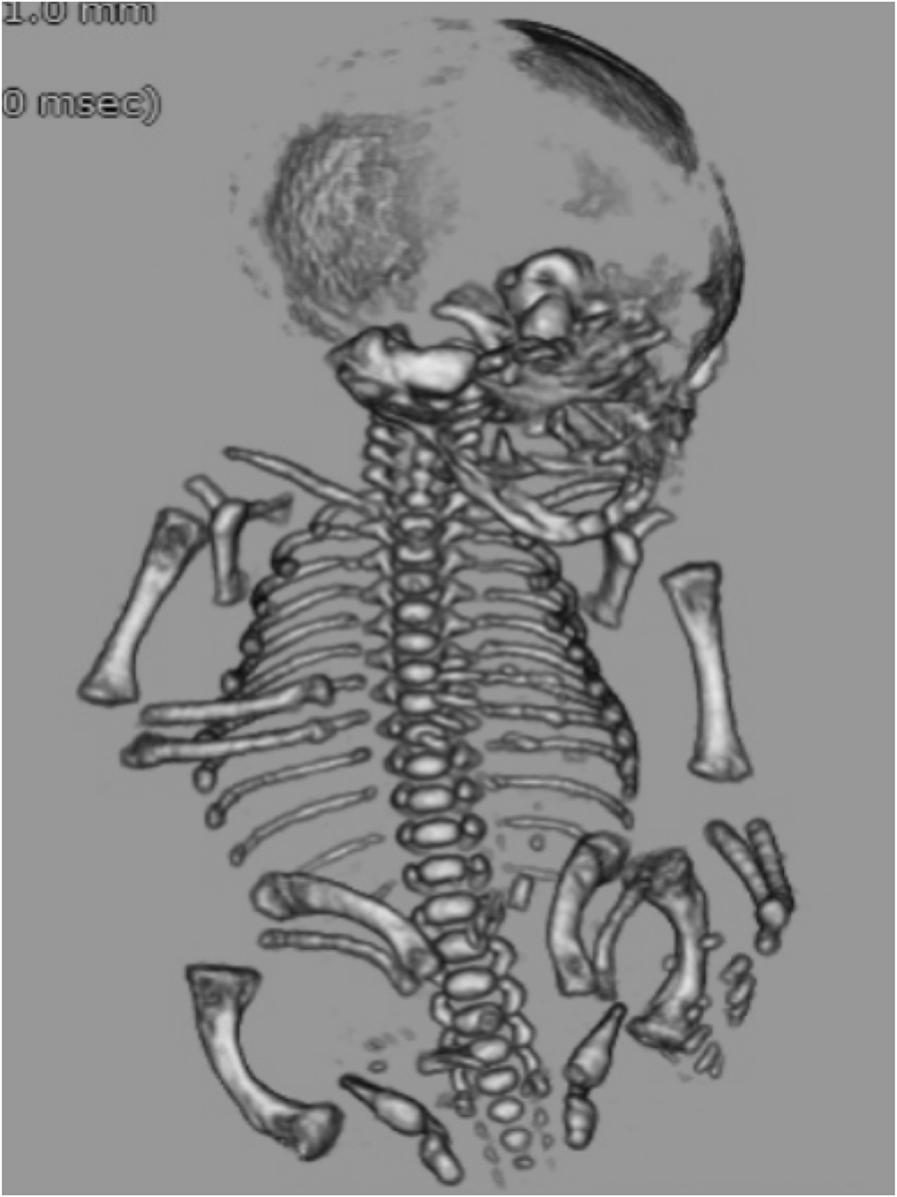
Fetal 3-dimensional computed tomography imaging of Infant 2: A case of osteogenesis imperfecta
